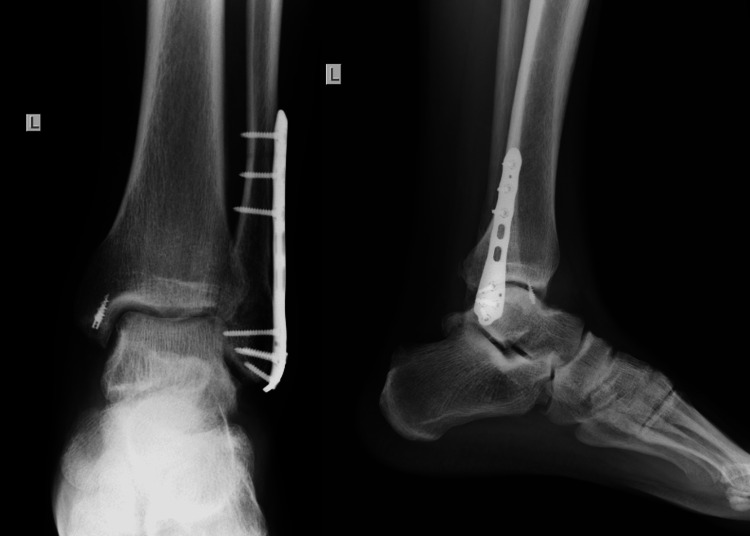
Three-month follow-up X-rays. Radiological examination in three-month follow-up.
